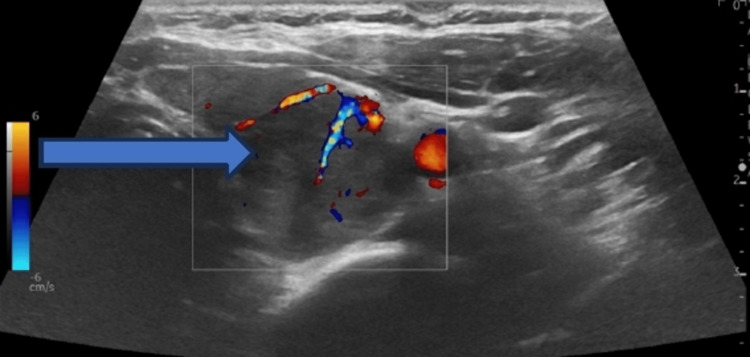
Color Doppler ultrasound scan shows parathyroid adenoma (pointed arrow)
